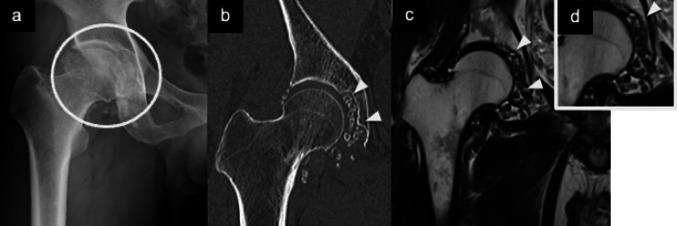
Synovial chondromatosis. Plain radiograph (a) and coronal CT (b) show multiple round and speckled calcifications (arrowheads) surrounding the proximal aspect of the right femur. Coronal T2-weighted MR images (c, d) demonstrate multiple low-signal foci (arrowheads), some with central high signal intensity, suggestive of fatty marrow within calcified nodules
